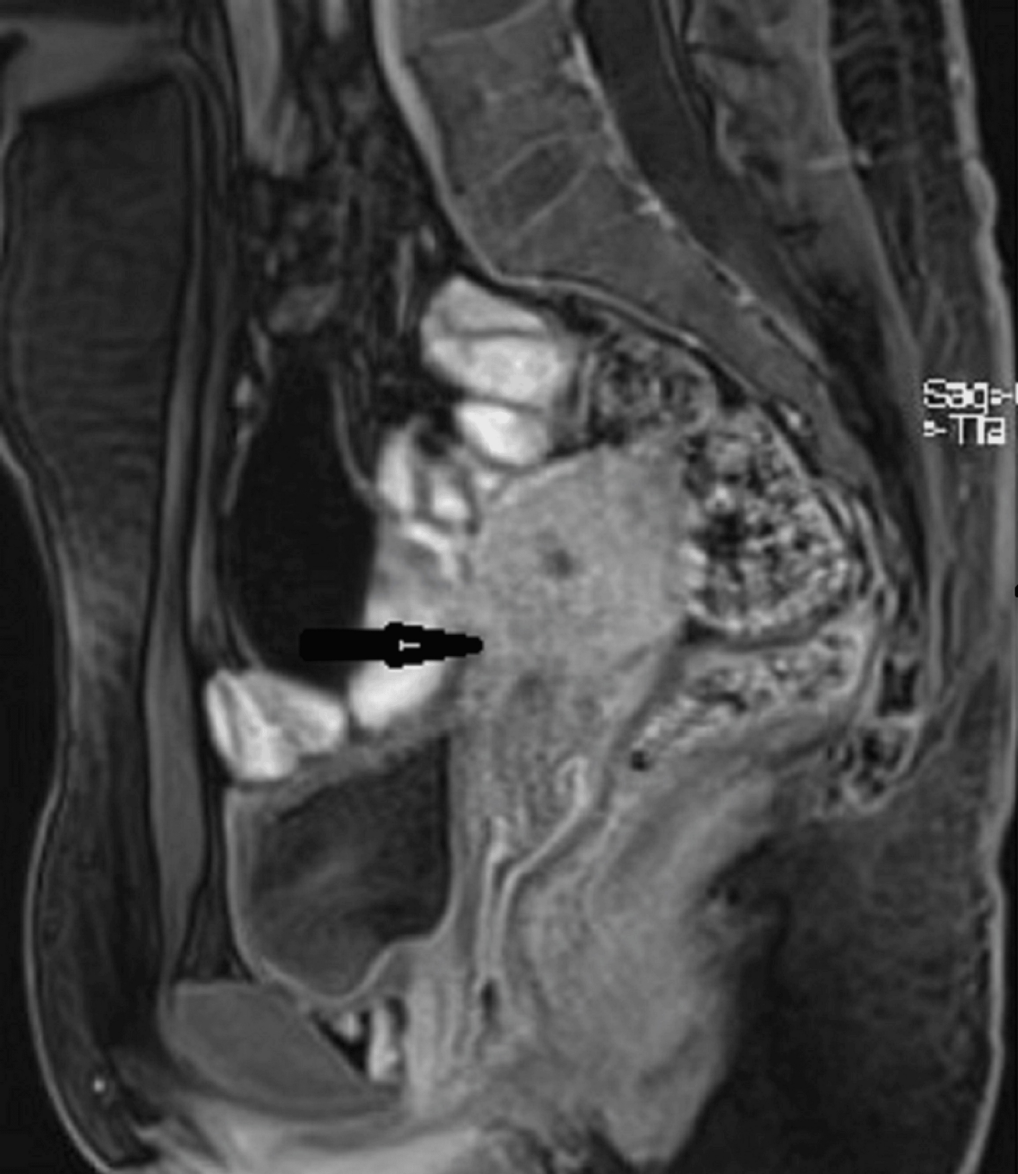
The junctional zone of the cavity thickened (13 mm), and the endomyometrial interface lacked clarity. The size and form of the major uterine cavity were normal, and the cornua were both clearly visible (arrow), ruling out the ultrasound diagnosis of a primitive horn.
